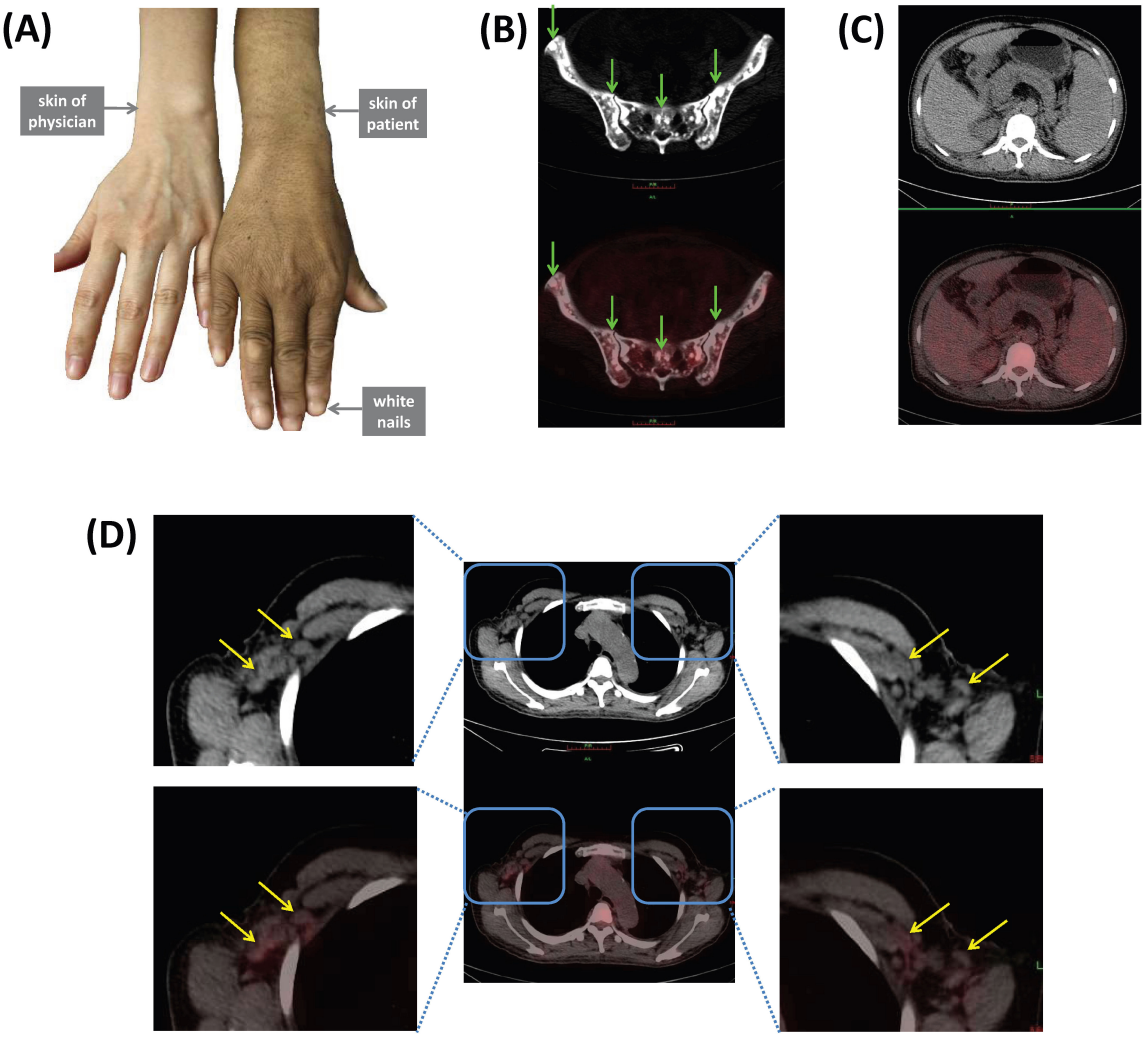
Representative manifestations of POEMS syndrome. (A) Skin changes including hyperpigmentation and white nails. ^18^F-FDG PET/CT scanning revealed (B) diffused osteosclerosis of pelvis (green arrows), (C) splenomegaly and hepatomegaly, (D) axillary fossa lymphadenectasis (yellow arrows).
